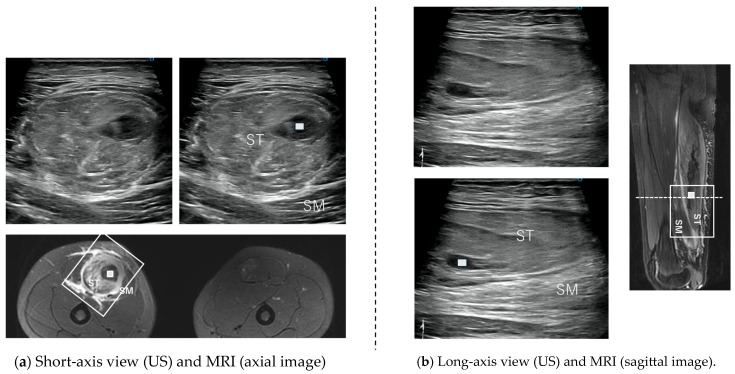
The US findings of a distal intermuscular tear of the ST/MRI condition: T2-weighted image with fat suppression; (a) there is a diffuse high-echo area of edema around the hematoma (white square). This section is distal to the raphe (dashed line in sagittal image); (b) there is a hematoma and diffuse edema due to the tear in the ST. There is SM in the deeper layers, and the boundary with the shallow ST is clear.
